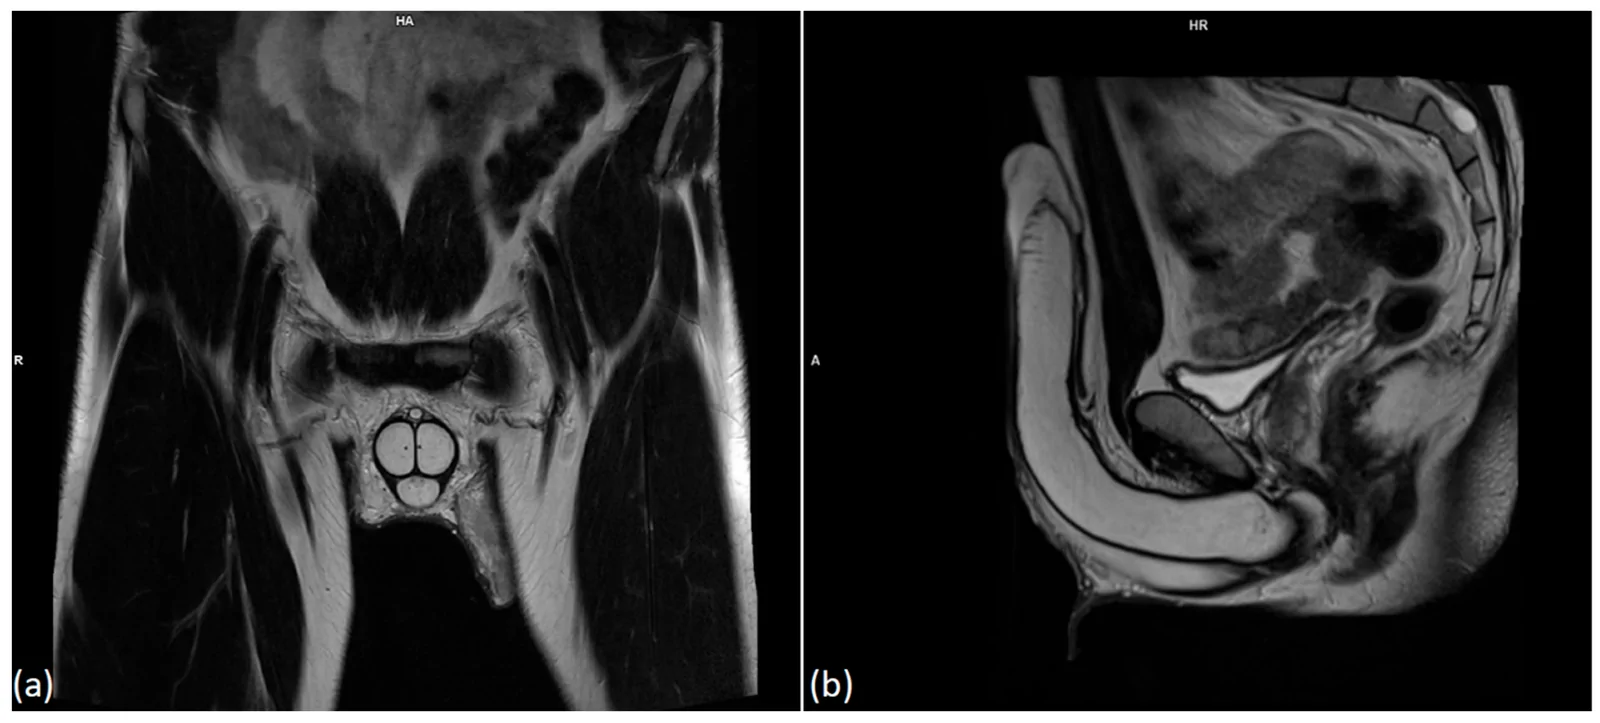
Normal penile anatomy on T2-weighted MRI (artificially induced erection): (a) coronal and (b) sagittal images delineating the penile structures.
